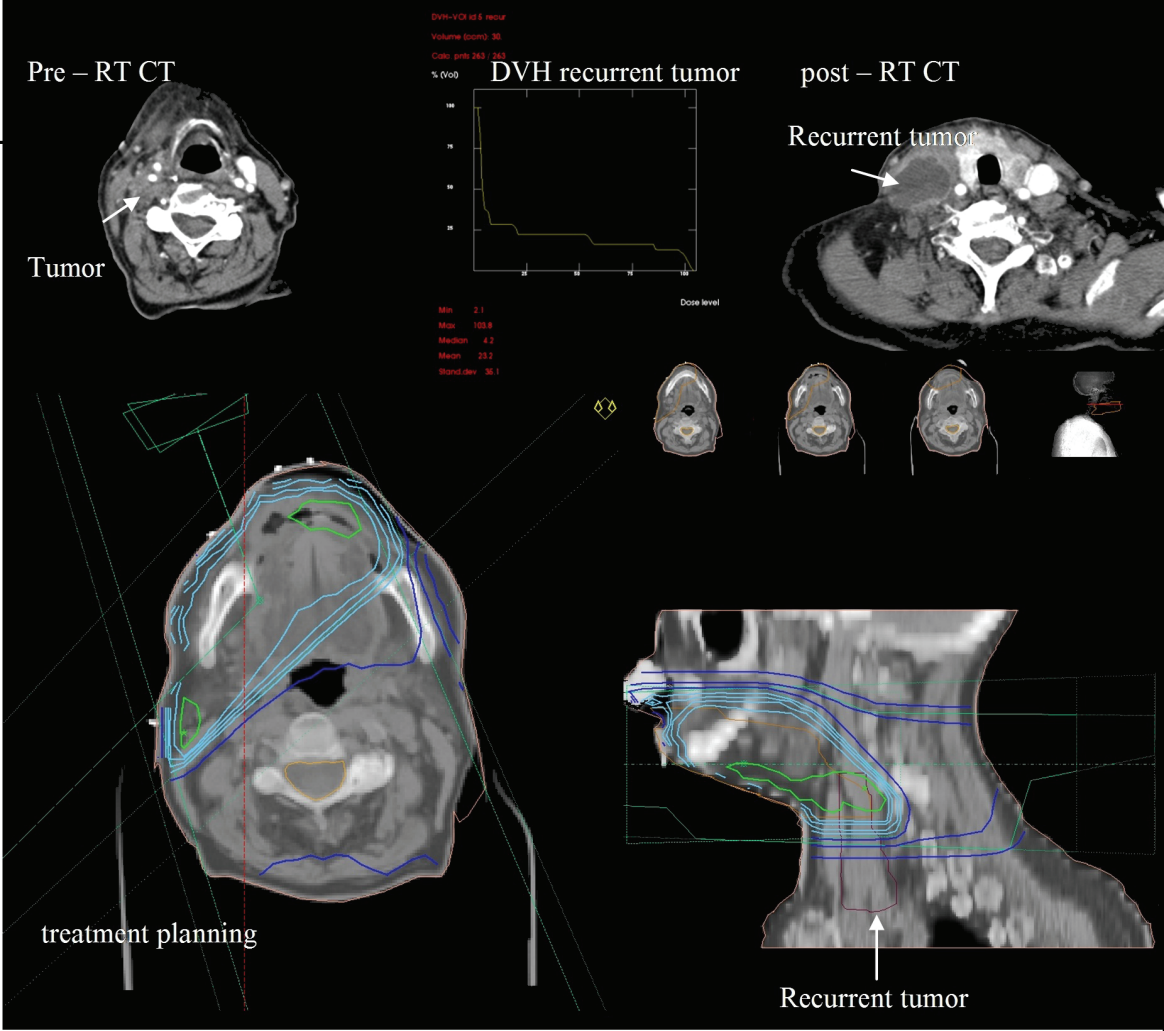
Reconstruction of site of tumour recurrence on pretreatment planning CT and DVH analysis to determine the site of recurrence in relationship to treatment volume: An example of out-of-field recurrent tumour in a patient with T2N1 oral cavity tumor(patient-5).
